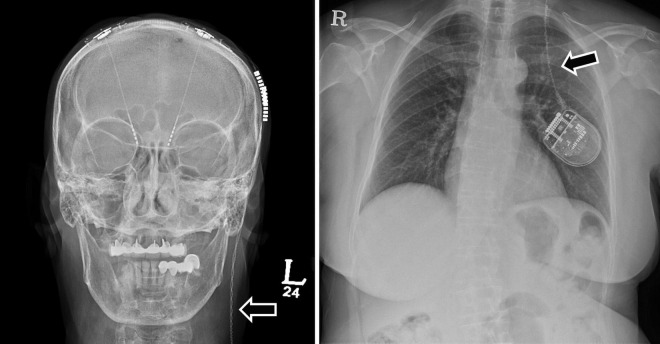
A 70-year-old woman with Parkinson’s disease had a broken extension wire due to Twiddler’s syndrome 3 months after primary DBS surgery. The skull and chest X-rays demonstrate twisted and broken extension wires due to Twiddler’s syndrome (arrows). The patient underwent revision surgery to replace the extension wire and perform multiple firm internal pulse generator anchor sutures. DBS, deep brain stimulation.
